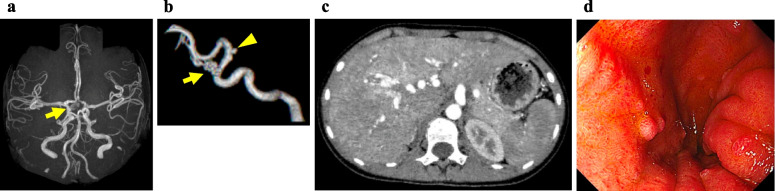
Brain magnetic resonance angiography showing two cerebral aneurysms, which are located at the right internal carotid–ophthalmic (arrow) and right internal carotid–anterior choroidal (arrowhead) arteries (a, b). Contrast-enhanced abdominal computed tomography showing hepatic arteriovenous malformations (c). Gastroenterological endoscopy showed diffuse polyps in the duodenum (d)
